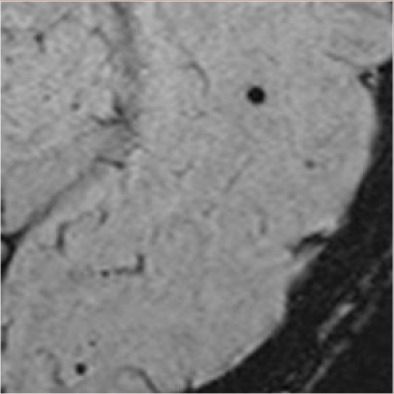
White matter hyperintensity	Increased signal on T2-weighted and FLAIR MRI. Mainly located in white matter; subcortical hyperintensities in deep gray matter and brainstem are not included in WMHs.	Gliosis, demyelination, blood brain barrier impairment. Associated with abnormalities of small cerebral arteries.
